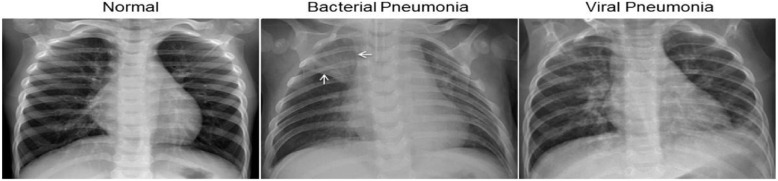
Comparison of normal lung X-ray and pneumonia X-ray photos
